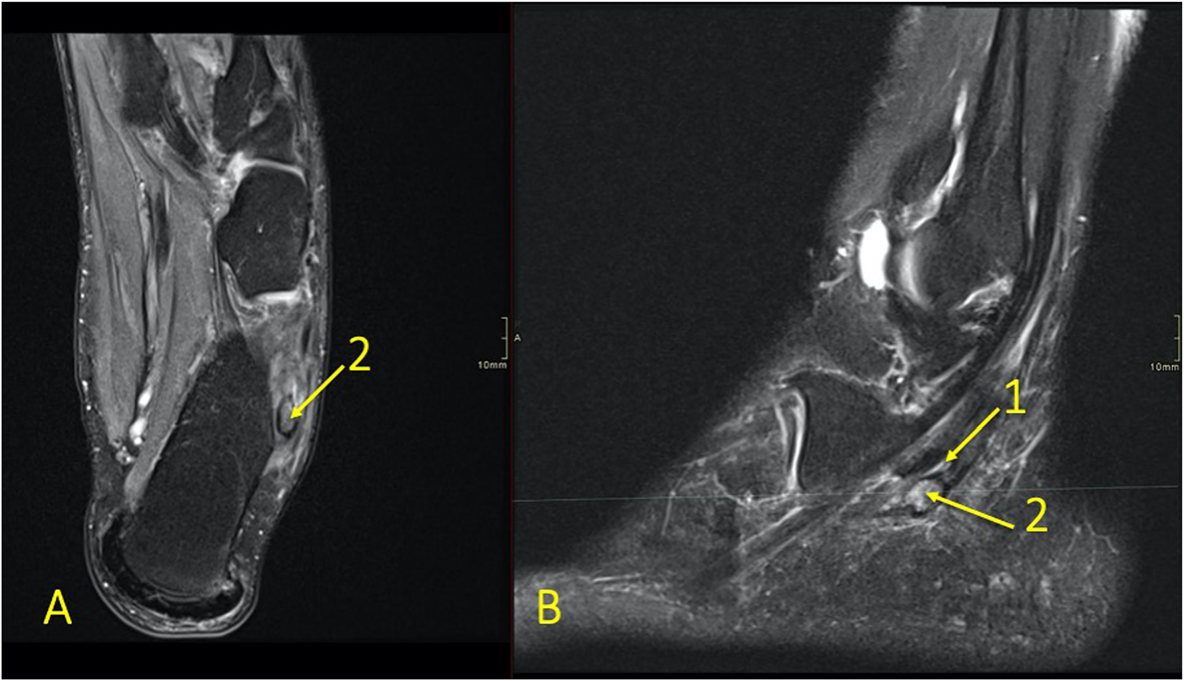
A 32-year-old handball player presented after a match with lateral ankle pain and a suspected lateral malleolar fracture. (1) MRI revealed a split rupture of the peroneus longus tendon (2) at the level of an os peroneum with BME
